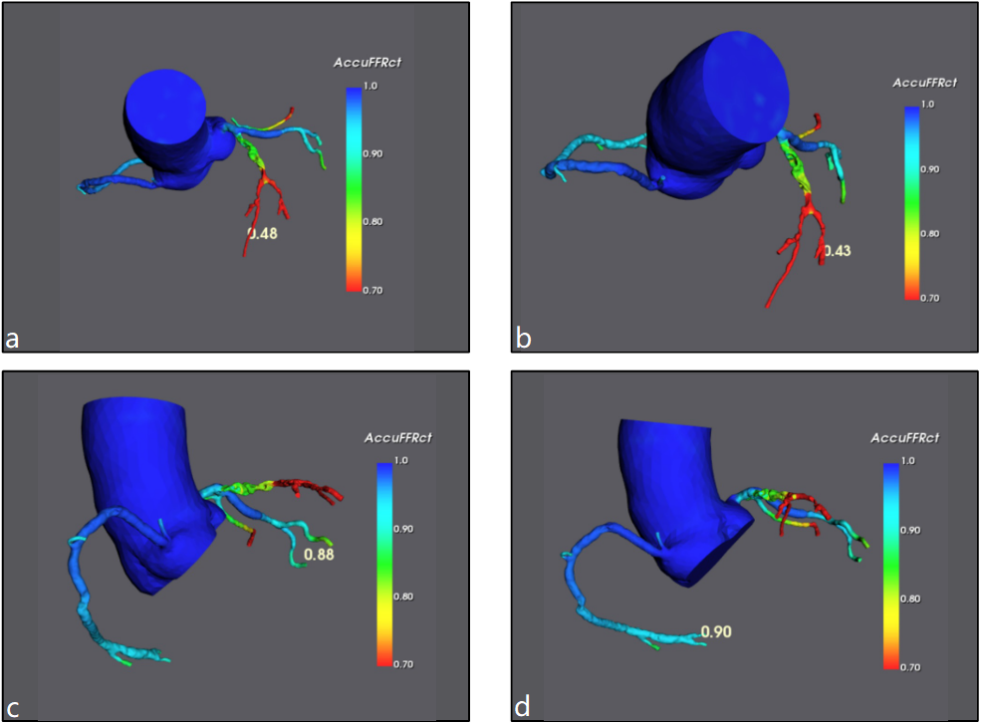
Functional analysis of coronary computed tomography
